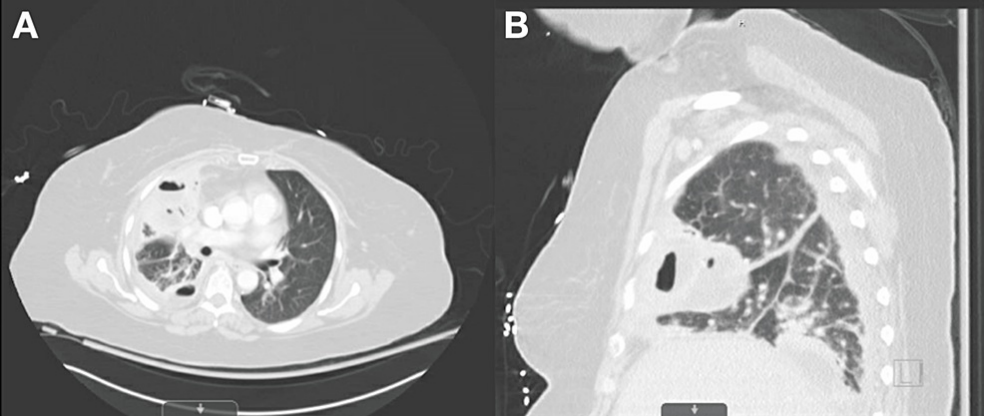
Computed tomography of the chest with intravenous contrast. Computed tomography with intravenous contrast after two rounds of intrapleural thrombolysis. (A) Axial and (B) coronal views showed a loculated fluid collection in the right oblique fissure between the right middle lobe and the right lower lobe. Additional smaller pleural loculations are redemonstrated abutting the right anterior chest wall with a small loculated hydropneumothorax.
